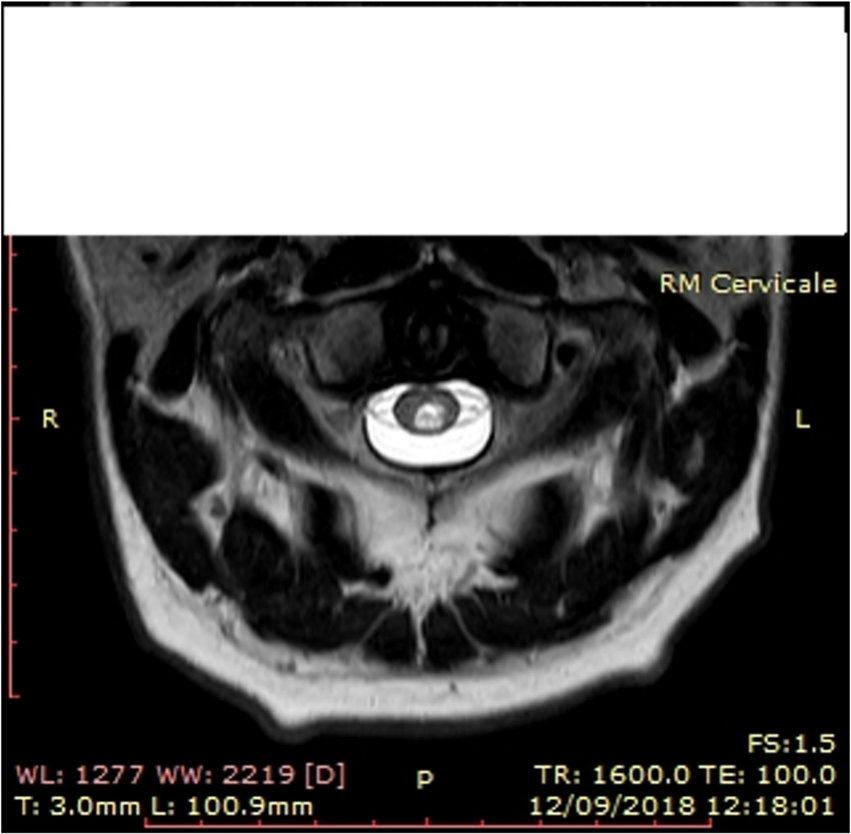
Cervical MRI. T1-weighted cervical MRI scan showing the spinal cord lesion in the medial posterior axial and sagittal C1 metamer.
